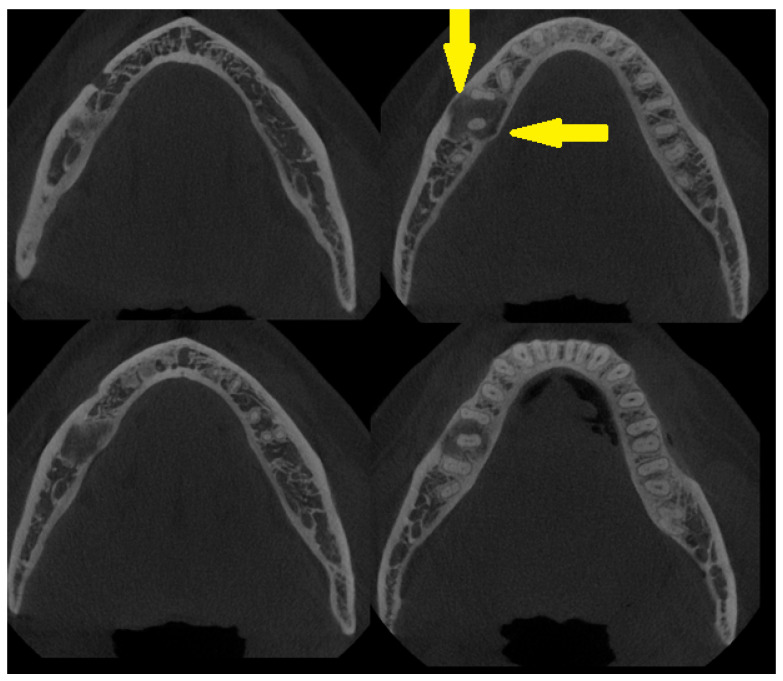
An axial CBCT view of the lesion—cortical swelling is barely noticeable; however, thinning of the buccal cortical plate can be seen along with totally intact roots of the first molar and second right premolar teeth (yellow arrows). The course of the mandibular canal was not disturbed, and clinically, no neurological symptoms from the inferior alveolar nerve were present (IAN). Because the lesion surrounded the first right molar and had some atypical bone borders (without tooth mobility, pain, or abnormal response to cold stimulus), and the lesion itself had a cloudy appearance without any clear signs of calcifications characteristic of fibrous dysplasia (DF) or any of the three growth/maturation stages characteristic of a COD lesion (cemento-osseous dysplasia), a decision was made to conduct a biopsy [4,5]. From a surgical point of view, COD small lesions might be observed clinically or radiologically or, in rare cases, scheduled for an incisional biopsy to remove and evaluate them. Some serious clinical implications start to arise when the lesion becomes larger and exhibits a non-characteristic radiographic appearance on CBCT. Due to the absence of general and local symptoms and the accidental discovery of the bone lesion, after the CBCT, a biopsy was scheduled, and the diagnosis of OSF was confirmed. On the other hand, because of the similarities among COD, DF, and OSF, in classic, non-growing, and self-limiting bone lesions, observation combined with tooth vitality testing is sufficient for the local control of the disease. Radiological signs like root resorption, cortical spread, bone asymmetry, and others might require some type of surgical intervention.
